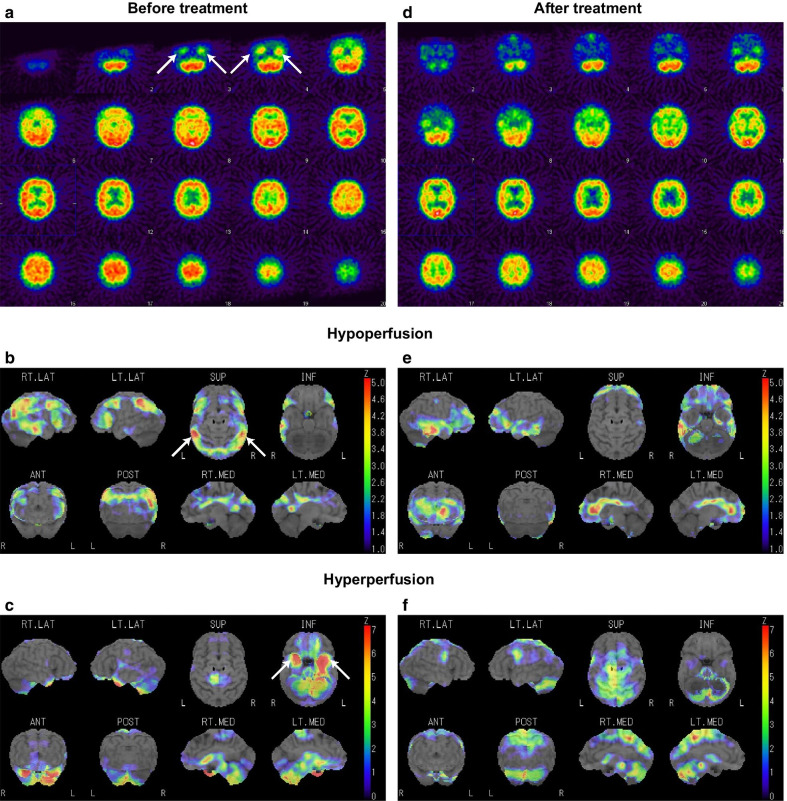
SPECT findings. a-c: The initial IMP-SPECT (1 week after admission). Before treatment, the abnormal bilateral hyperperfusion of the medial temporal lobes (arrows in a and c), as well as the bilateral hypoperfusion in the parietal regions (arrows in b), were observed. d-f: Follow-up IMP-SPECT (2 months after admission). After treatment, these abnormalities were resolved
